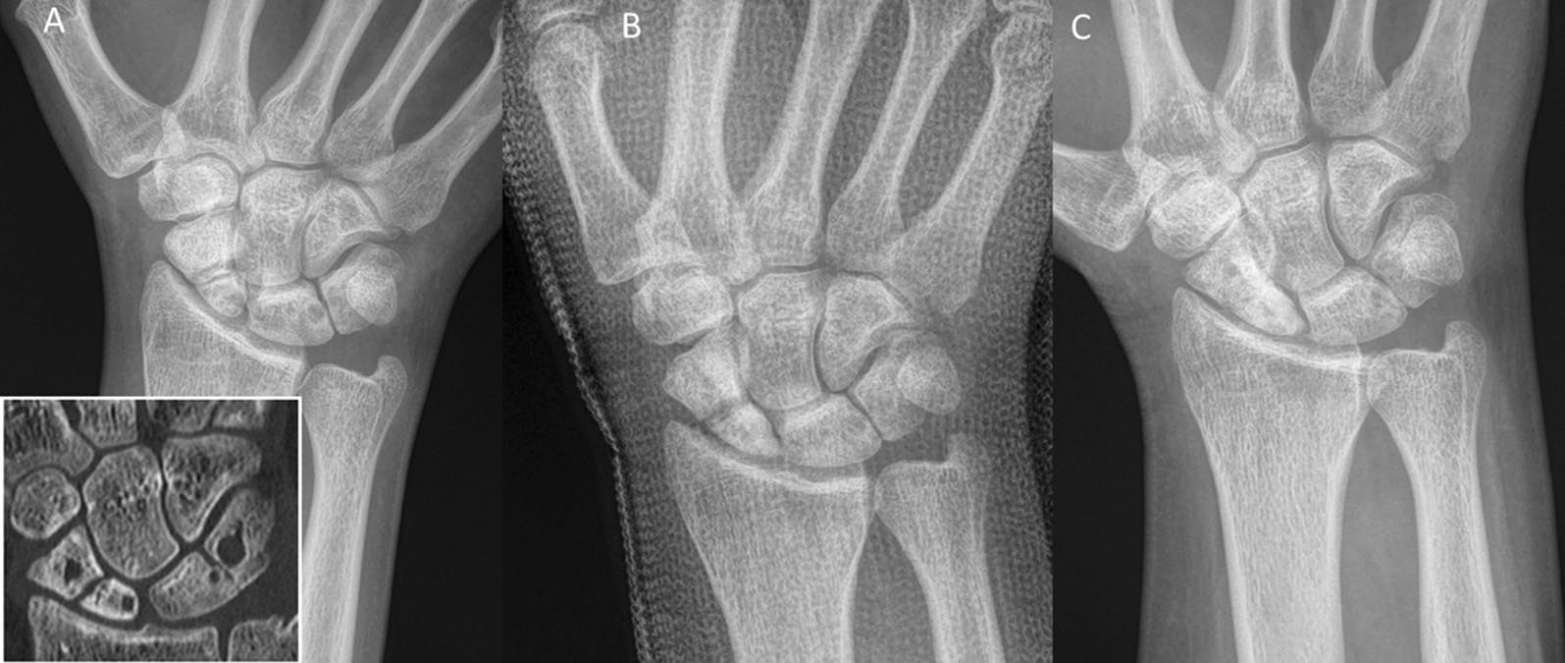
Example of cysts pre-surgery. Pseudarthrosis patient with a fracture in the middle third. A Pre-surgery.* Inset*: CT with good visibility of the cysts. B 1-day post-surgery. C 17 months post-surgery; no cysts are visible
